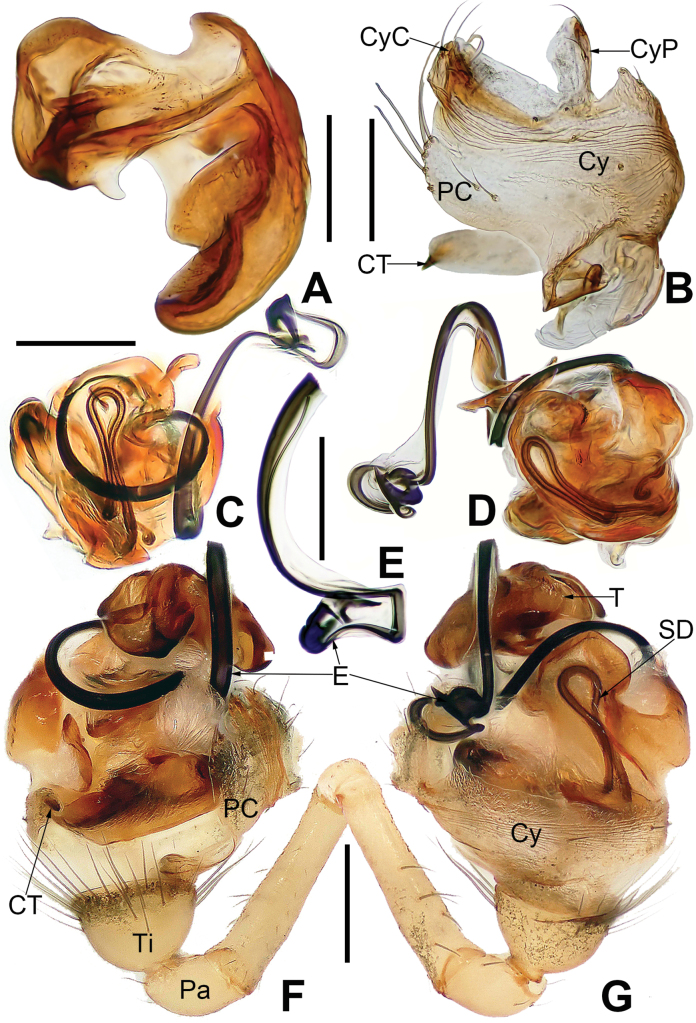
Microdipoenashenyang* sp. nov. from Liaoning of China A conductor, dorsal B cymbium, ventral C bulbus with conductor removed, prolateral D bulbus with conductor removed, retrolateral E embolic end, apical-lateral F male palp, prolateral G male palp retrolateral. Abbreviations: CT = cymbial tooth, Cy = cymbium, CyC = cymbial conductor, CyP = cymbial process, E = embolus, Pa = patella, PC = paracymbium, SD = spermatic duct, T = tegulum, Ti = tibia. Scale bars: 0.10 mm (A, B, E–G); 0.20 mm (C, D).
